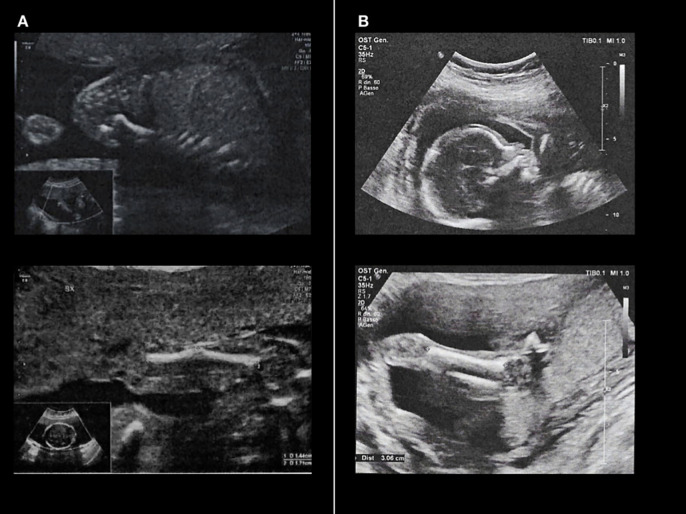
Fetal ultrasound of the two siblings of Family 2. (A) shows the images of the first fetus, showing multiple bone deformities and impaired bone mineralization. (B) displays images from the second pregnancy, showing a normal-appearing fetus with no skeletal deformities despite identical genotype.
